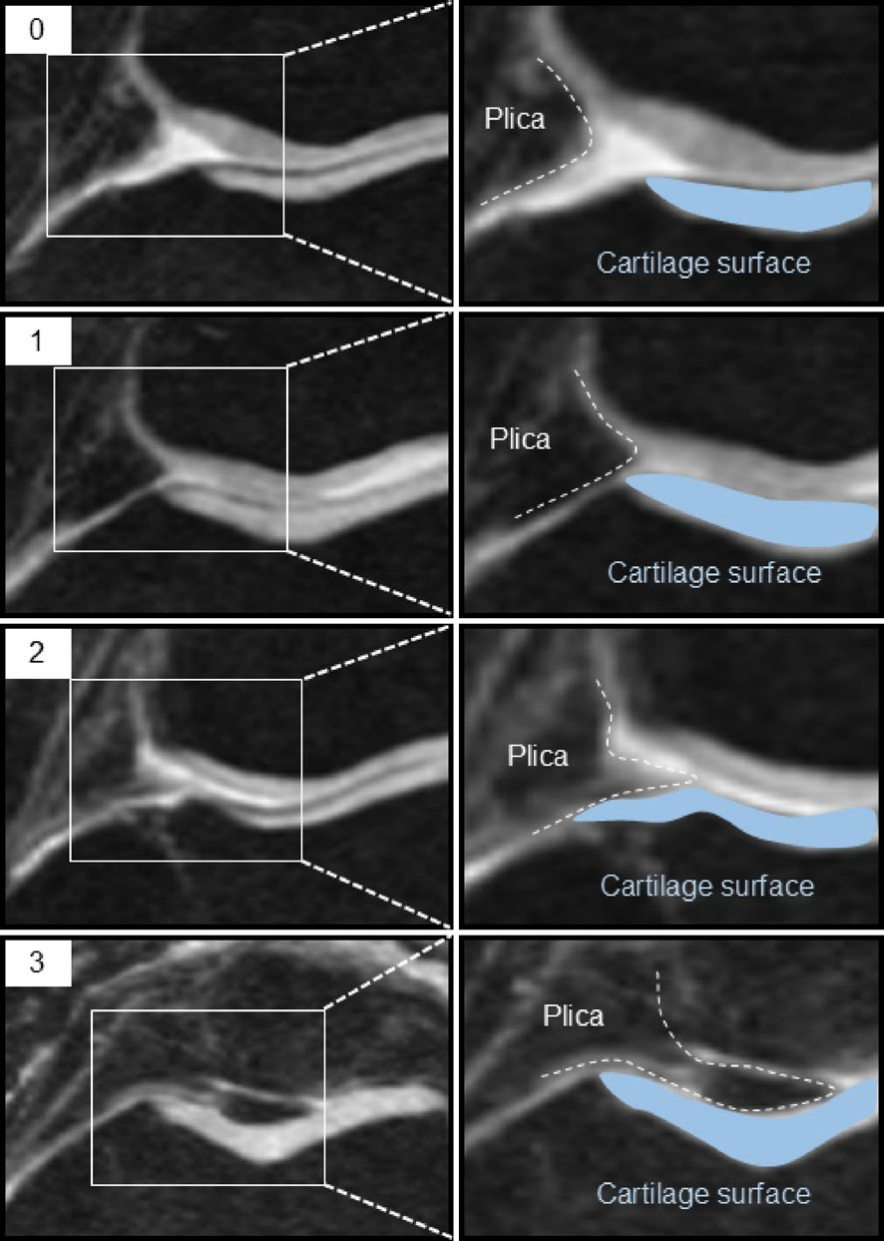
Severity of medial plica on MRI. Grade 0, no obvious plica; grade 1, cord-like elevation in the synovial wall; grade 2, a shelflike appearance but no coverage of the anterior surface of the medial trochlea; grade 3, a large shelf-like appearance and coverage of the anterior surface of the medial trochlea
